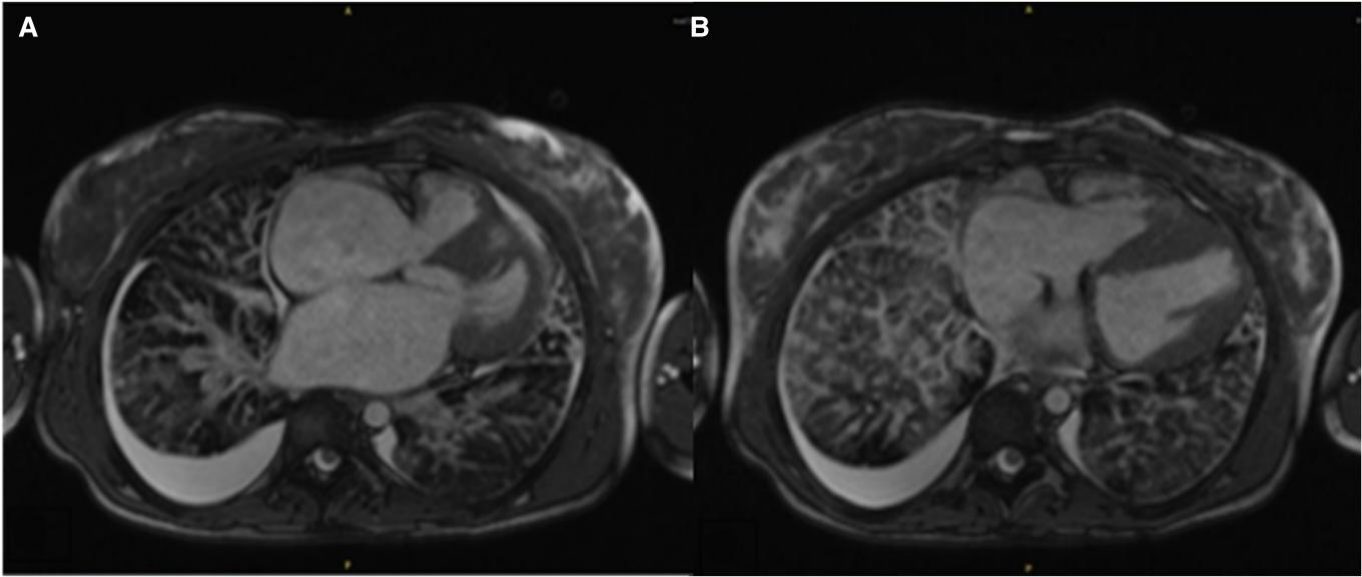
(*A*) Axial cardiac magnetic resonance imaging using steady-state free precession sequence demonstrating qualitatively severe biatrial dilation with reduced ventricular volumes (left ventricular end-diastolic volume index 65 mL/m^2^ and right ventricular end-diastolic volume index 71 mL/m^2^) and preserved biventricular function (left ventricular ejection fraction 63% and right ventricular ejection fraction 66%) in keeping with restrictive cardiomyopathy. (*B*) Associated findings include thickened interlobar fissures, interlobular septal thickening accompanied by areas of pulmonary lymphangiectasia and bilateral pleural effusions (right > left). These features reflect systemic venous and lymphatic congestion secondary to impaired diastolic filling.
